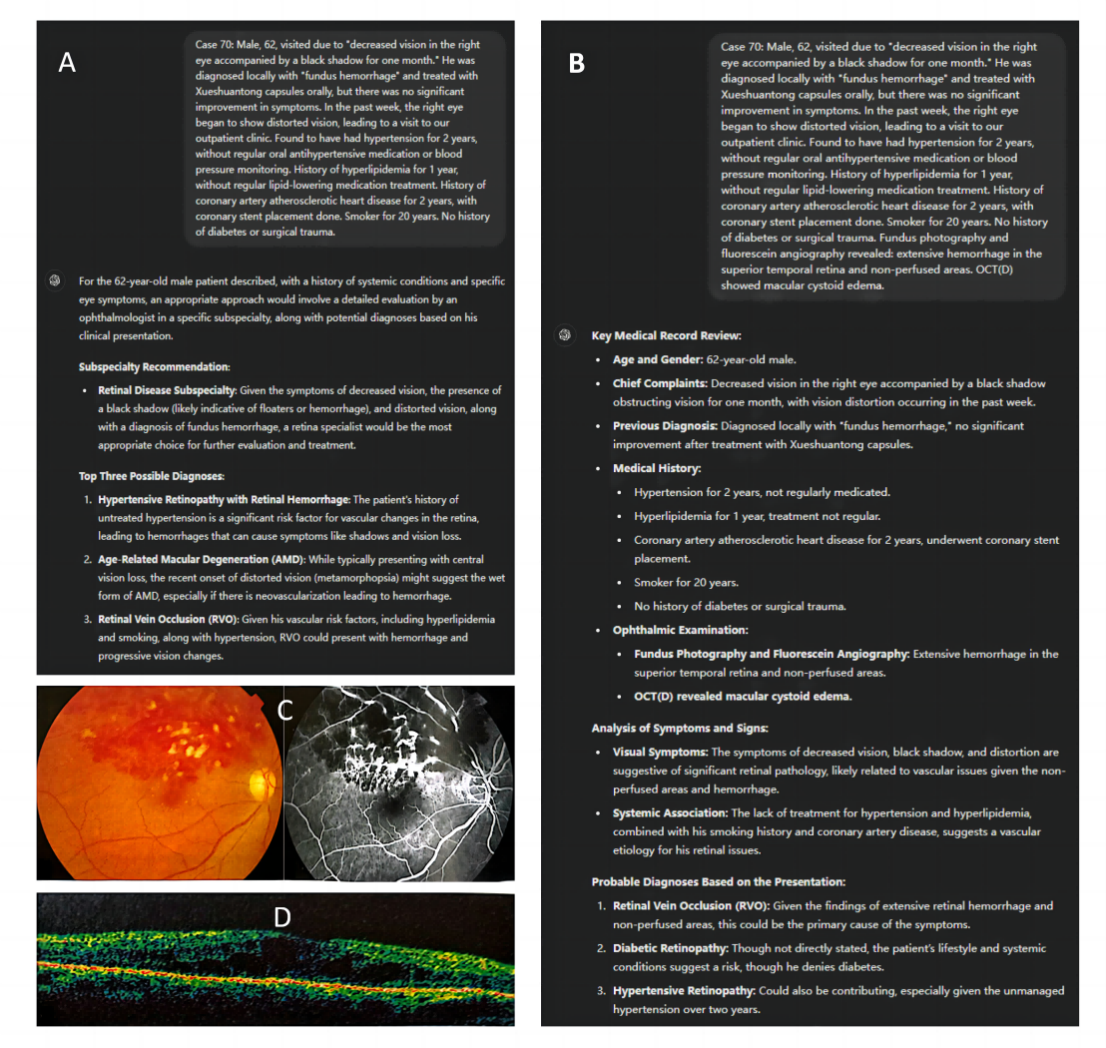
Example profile 70: interaction with and responses of the GPT-4.0 chatbot. (A) When provided with Hx information, GPT-4.0 correctly recommended the ophthalmic subspecialty of “retinal diseases.” (B) When provided with Hx+Ex information, GPT-4.0 gave the top 3 diagnostic suggestions and accurately identified “retinal vein occlusion” as the top-ranked diagnosis. (C) Fundus photography and fluorescein angiography. (D) OCT imaging. (C) and (D) were presented to ChatGPT as textual descriptions of the examination results. Hx: patient with history; Hx+Ex: patient with history and examination; OCT: orbital computed tomography.
